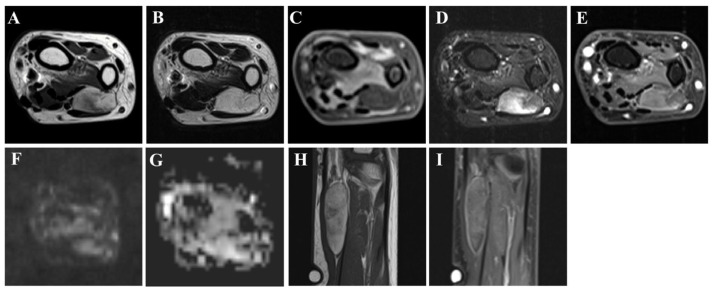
On magnetic resonance imaging (MRI), the lesion measured 35 × 20 × 12 mm and was located in the intermuscular space deep to the flexor carpi ulnaris. It contained macroscopic fat that was suppressed on fat-suppressed sequences, whereas its non-fatty components showed isointensity to muscle on axial (A) and sagittal (H) T1-weighted (3T; TR/TE = 600/13) and axial fat-suppressed T1-weighted images (C), as well as moderately high to high levels of signal intensity on axial T2-weighted (TR/TE = 4000/99) (B) and axial fat-suppressed T2-weighted images (D). Diffusion-weighted imaging (b = 1000) revealed mildly high signal intensity (F). The apparent diffusion coefficient within the lesion region of interest was 1.44711 × 10^−3^ mm^2^/s (G). The non-fatty components exhibited mild enhancement (E) axial and (I) sagittal after contrast administration, suggesting a fat-containing tumor with non-fatty components. The presence of non-fatty areas and mild enhancement made a simple lipoma unlikely. The lack of internal septa, small size, and high fat content made spindle cell lipoma (SCL) and hibernoma more likely than atypical spindle cell/pleomorphic lipomatous tumor (ASPLT) or atypical lipomatous tumor/well-differentiated liposarcoma (ALT/WDL) based on imaging findings. A preoperative biopsy was considered; however, due to the small size of the lesion, its deep location adjacent to the ulnar neurovascular bundle, and the potential risk of sampling error in a heterogeneous fat-containing tumor, complete excision was selected for both diagnostic and therapeutic purposes. Unlike in our case, even when malignancy is suspected, biopsy may be technically impossible in very small lesions. In such situations, even if the final diagnosis turns out to be a sarcoma, additional wide resection can be performed after the initial excisional biopsy [1]. A longitudinal incision was made along the ulnar aspect of the wrist, and the mass was identified deep to the flexor carpi ulnaris. Although the ulnar artery and ulnar nerve were in close proximity, they were easily preserved, and the tumor was removed en bloc.
